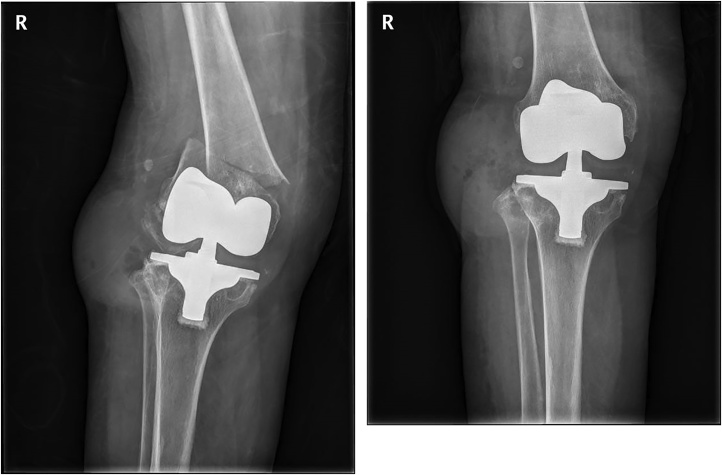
A) Radiograph showed a complete displaced fracture, which was not seen at visiting an outpatient clinic. (B) radiograph taken at outpatient clinic showed a huge soft tissue mass shadow on antero-lateral aspect of knee.
